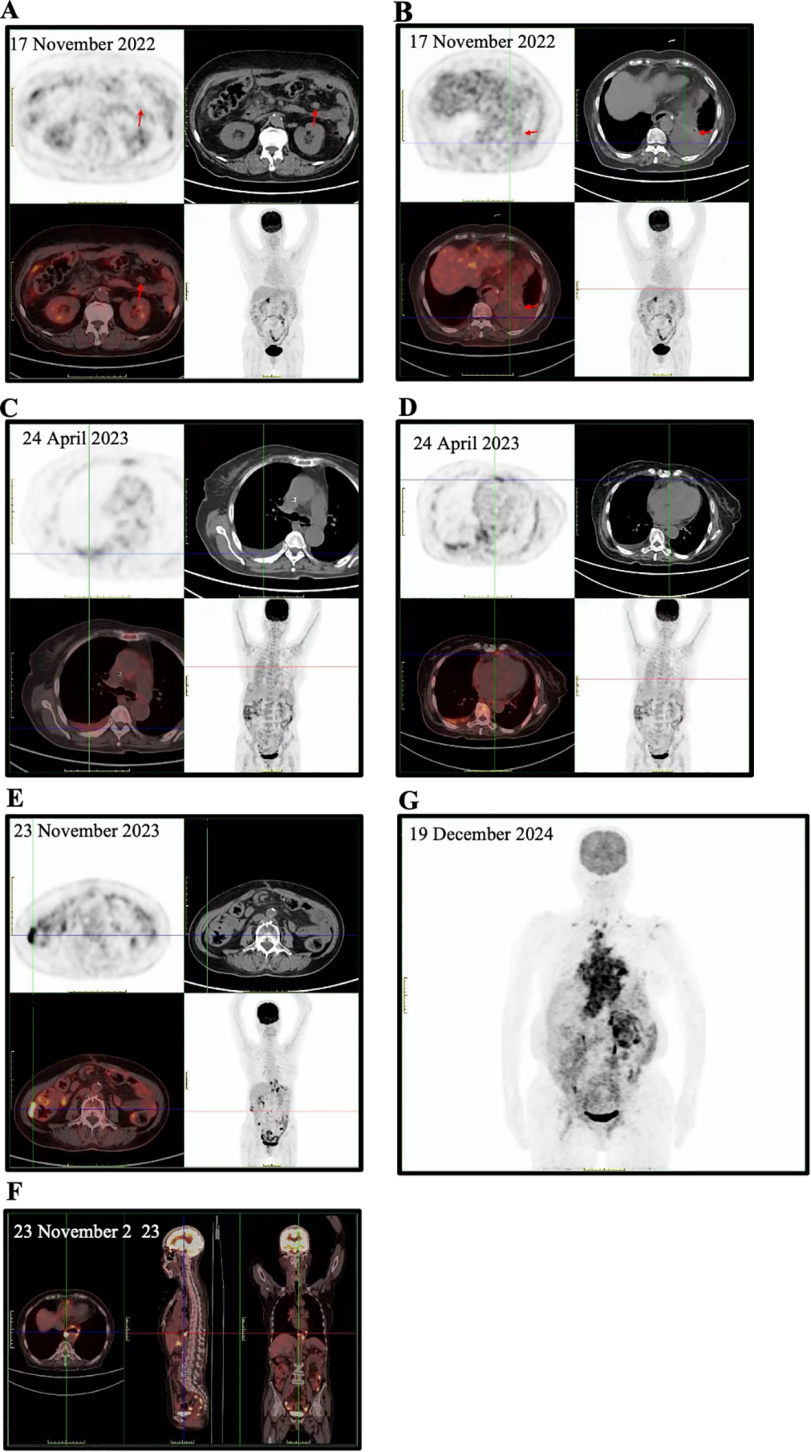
The results of four imaging examinations that documented changes in the patient’s condition. (A) PET/CT demonstrates a hypermetabolic nodule in the left abdominal cavity. (B) PET/CT reveals left-sided pleural effusion. (C–D) PET/CT shows increased metabolic activity within pleural and pericardial effusions. (E-F) PET/CT demonstrates newly identified heterogeneous thickening of the right colonic mesentery with increased FDG uptake, along with newly developed abnormal hypermetabolism at the distal esophageal anastomotic site. (G) PET/CT reveals multiple hypermetabolic lesions distributed throughout the body.
